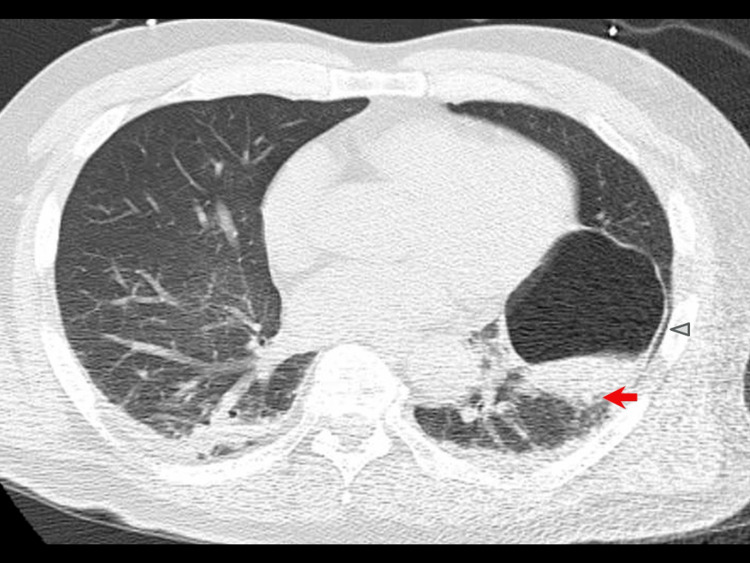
Chest CT on admission (Day 0). Chest CT on admission confirms a 7-cm thin-walled, fluid-filled emphysematous cyst located in the lingular segment of the left upper lobe (arrowhead). The dorsal wall of this cyst closely abuts the left inferior pulmonary vein (arrow).
